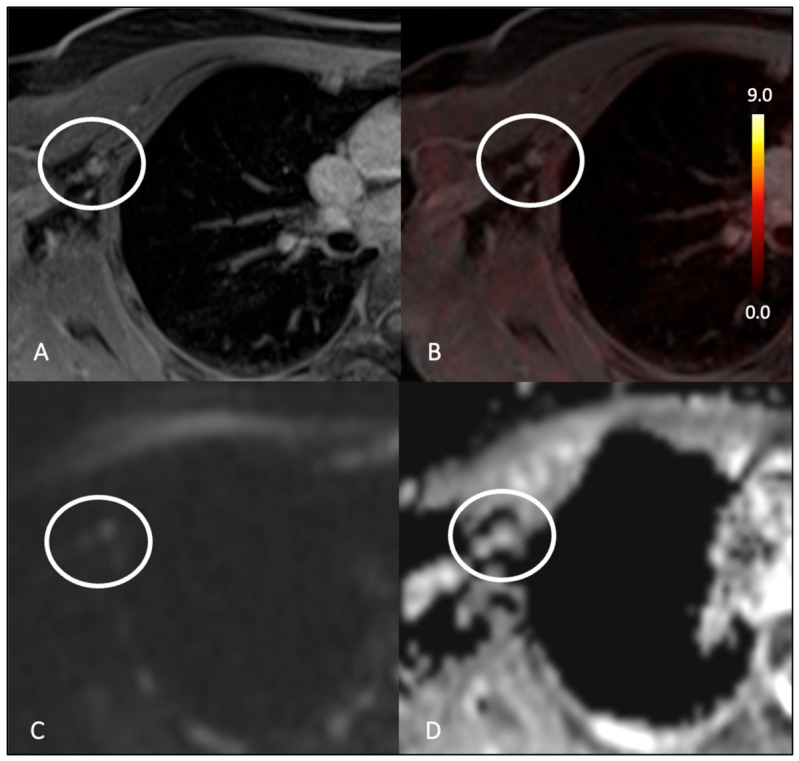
Right axillary benign lymph node in a breast cancer patient (tumor stage according to TNM classification: cT2 N0 M0). Gadolinium-enhanced T1 image (A) and fusion image of [^18^F]FDG-PET/MRI (B) showing no focal tracer signal (FDG−). Focal area of moderate signal intensity on b1000 DWI image (C) and with moderate ADC value (1.24 × 10^−3^ mm^2^/s) on the ADC map (D).
